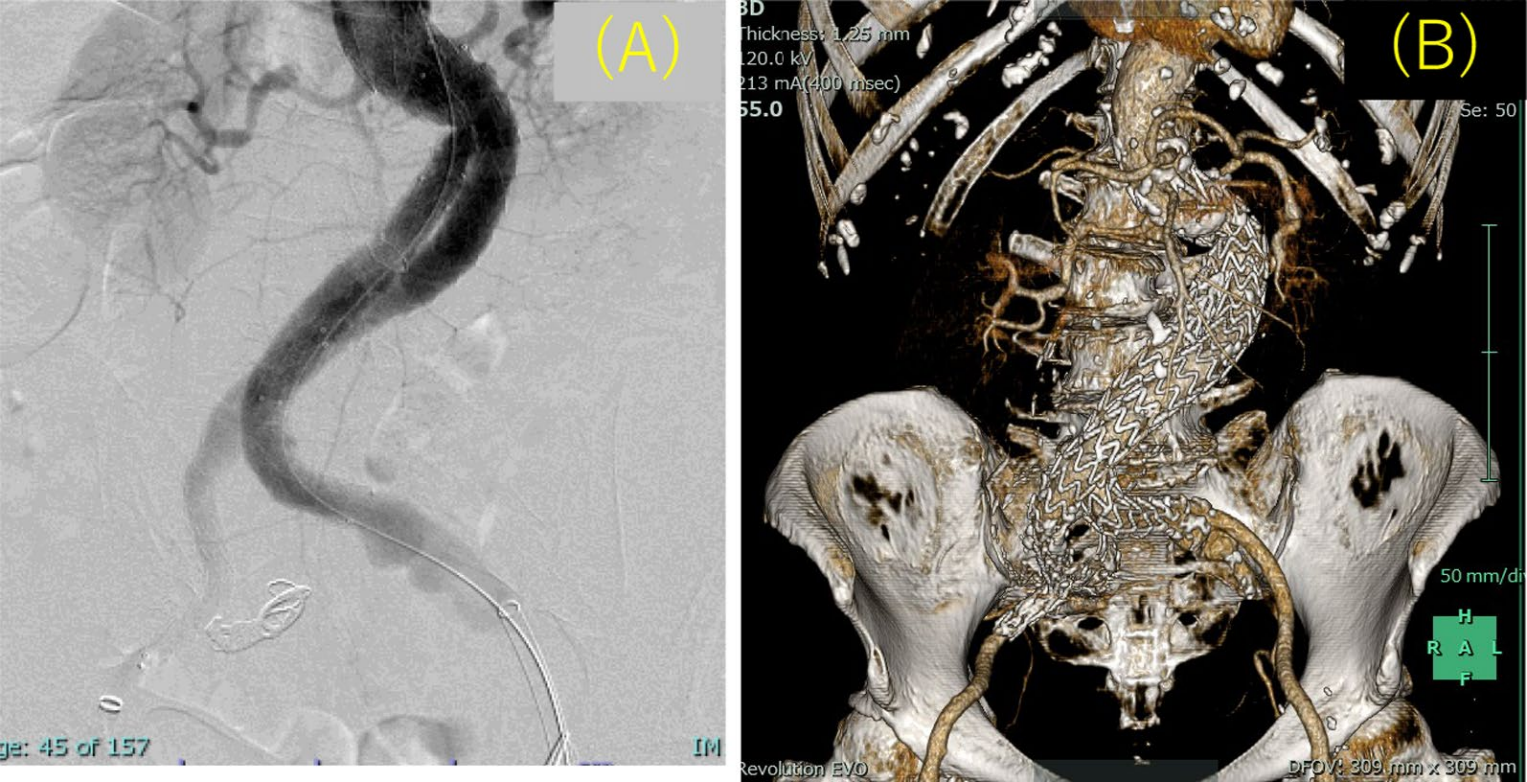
A Aortography after stent graft insertion. The abdominal aortic aneurysm and right common iliac artery aneurysm were excluded without any obvious endoleak, and the bilateral renal arteries were preserved. B Postoperative three-dimensional computed tomography findings
